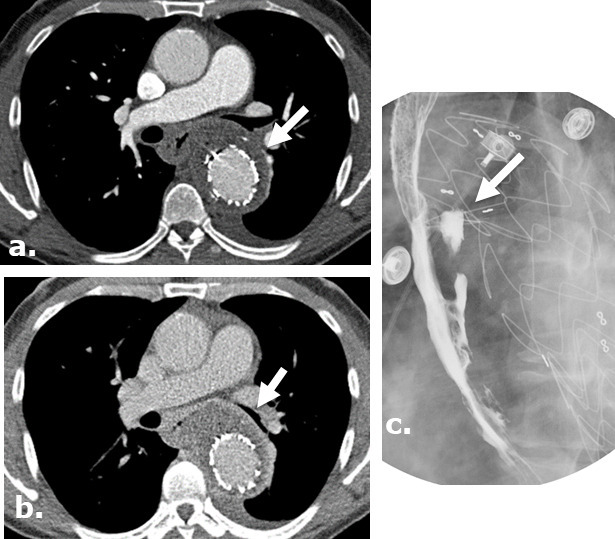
Aorto-esophageal fistula, leading to mediastinal abscess in a surgical case where Surgicel was not used. Arterial (a) and delayed (b) phases of CTA of the chest of a 56-year-old male demonstrate perigraft gas-containing fluid collection containing small gas bubbles. There is esophageal wall thickening and loss of the fat plane between the aorta and esophagus. A barium swallow study demonstrates extravasation of barium toward the aortic stent, consistent with aorto-esophageal fistula (c).). Patient was treated with antibiotics and G-tube placement.
